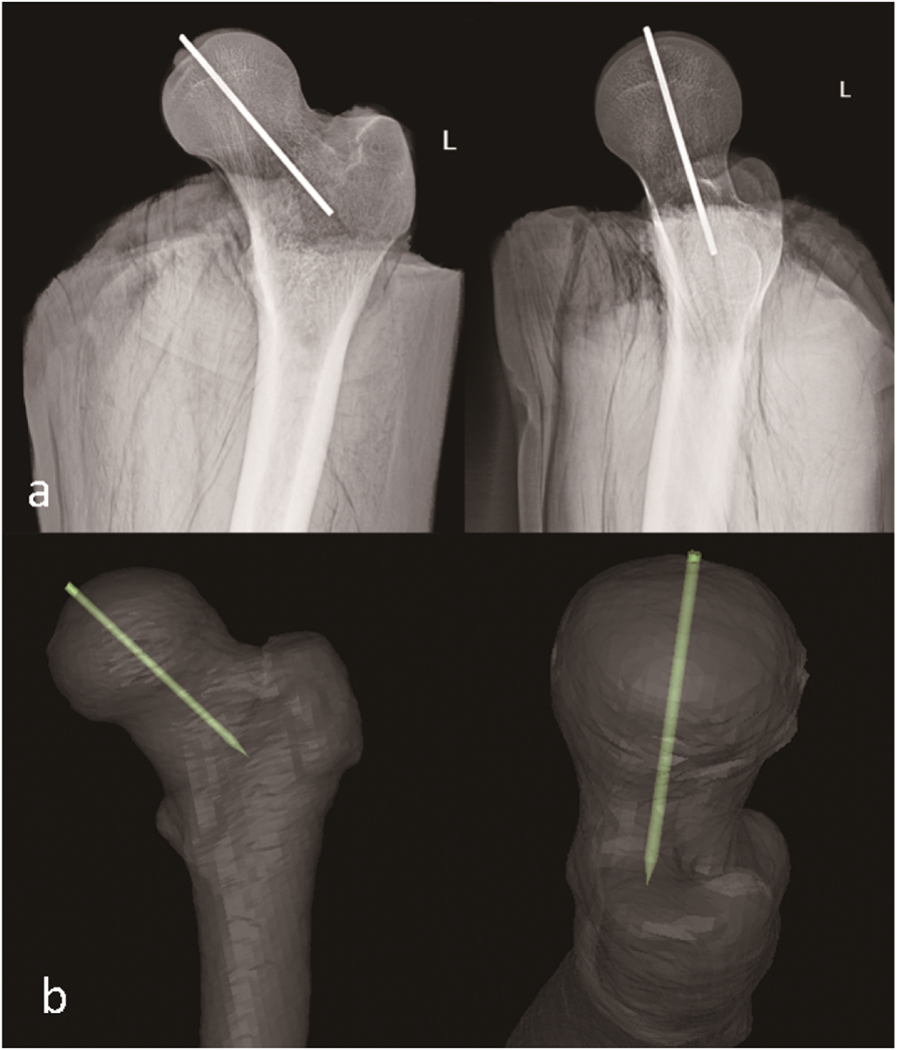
Postoperative x-rays and CT reconstruction show the perfect and accurate position of Kirschner wire. a Postoperative x-rays show the anteroposterior and lateral position of the Kirschner wire. b 3D reconstruction of postoperative CT scan shows the optimal postion of the Kirschner wire
